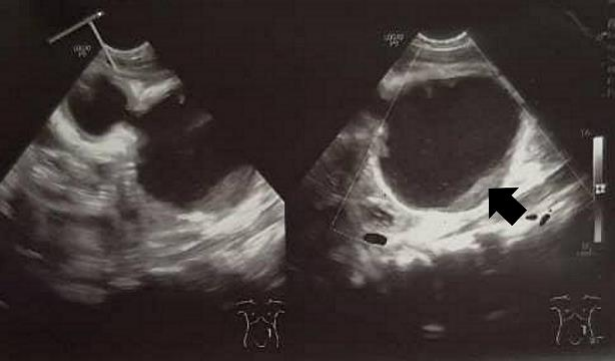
ultrasound imaging reported as duplication cyst with echogenic material within (arrow)
